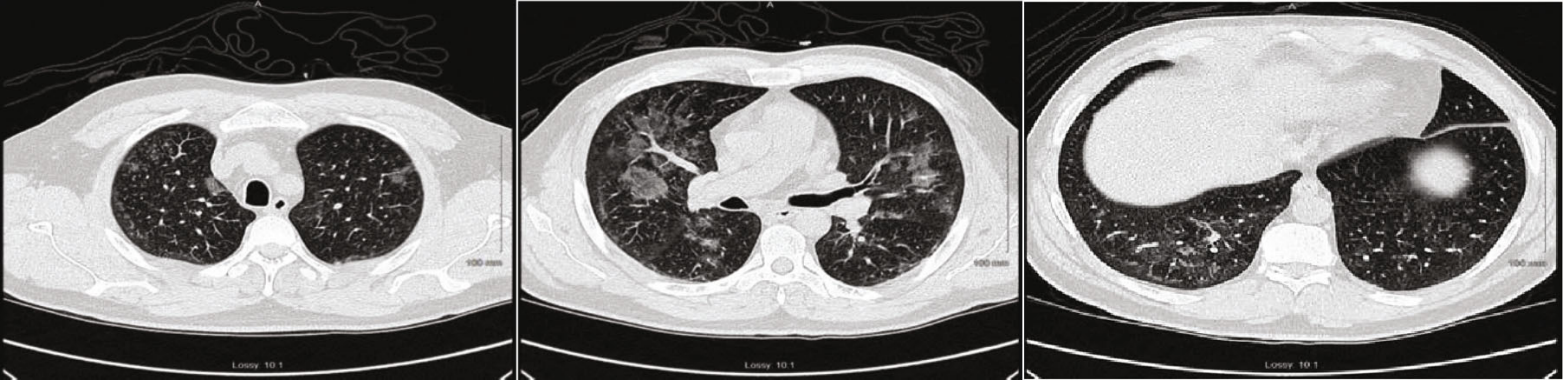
Chest CT showing nonspecific bilateral ground glass opacities.
